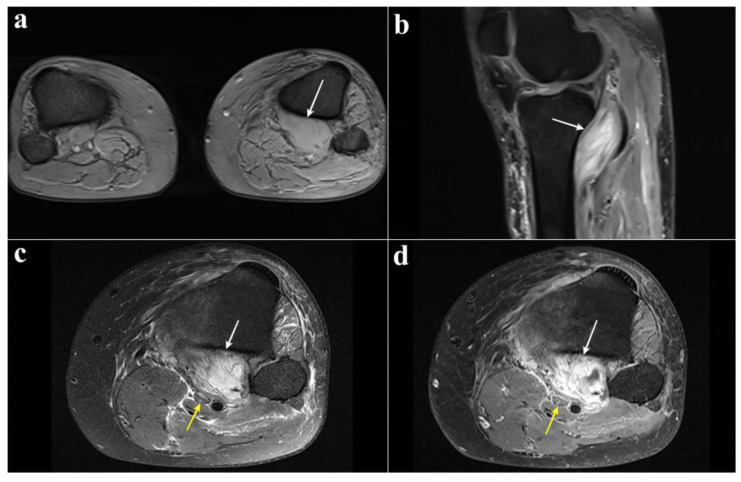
Axial T2-weighted MR images at the level of the proximal leg, comparing both right and left legs. There is a marked asymmetry of the popliteus muscles, with the left popliteus muscle (white arrow) appearing enlarged compared to the contralateral side on the right (a). Sagittal fat-suppressed proton density-weighted MR image of the left knee. The swollen popliteus muscle is visible (white arrow), exerting a mass effect with displacement of the popliteal vessels and the tibial nerve (b). Axial MR images of the proximal left leg. Fat-suppressed proton density-weighted sequence (c) and contrast-enhanced fat-suppressed T1-weighted sequence (d) demonstrating marked swelling and abnormal signal with diffuse enhancement of the popliteus muscle (white arrow) with posterior displacement of the tibial nerve (yellow arrow).
